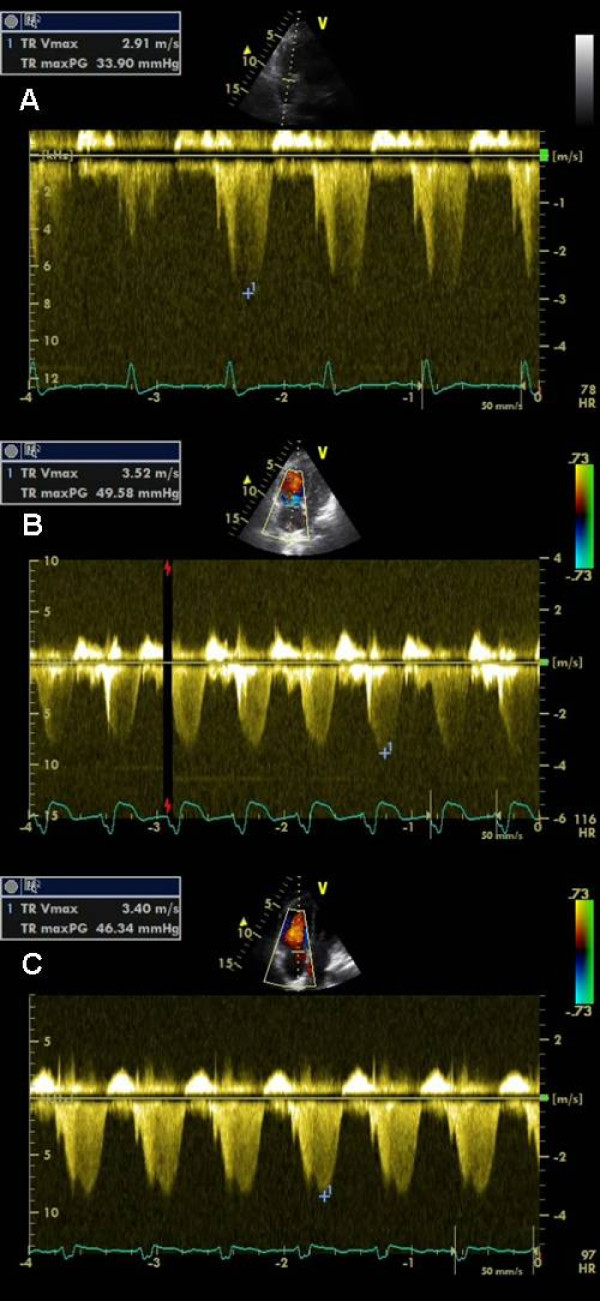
Pulmonary artery pressure (PAP). Continuous-wave Doppler echocardiography was used to estimate PAP: (A) on admission, (B) during the acute phase of pulmonary embolism with right heart thrombus, and (C) on day 2 after thrombolysis.
